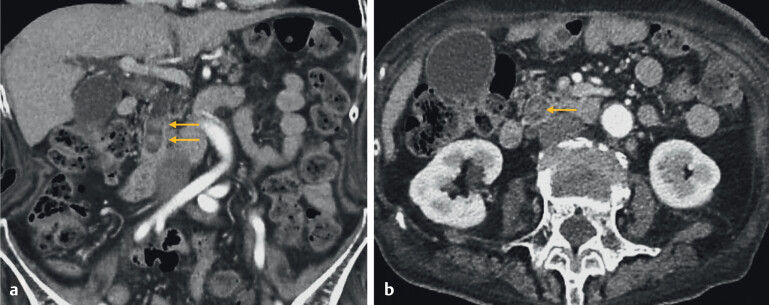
Computed tomography showing multiple common bile duct stones
